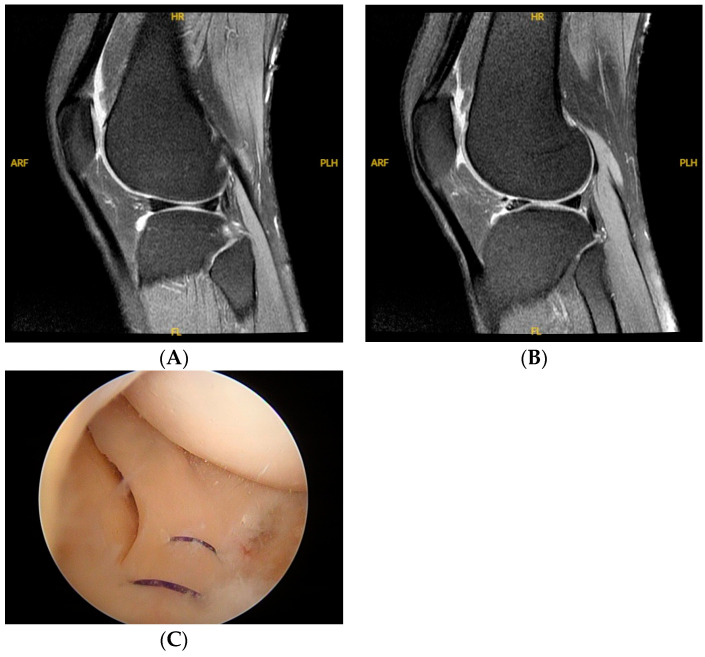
Preoperative knee magnetic resonance images of a 59-year-old female patient, and the arthroscopic image following meniscal stabilization. (A). The image shows the central region of the knee, which appears normal. The meniscus structure is intact, with uniform signal intensity and no abnormal high-signal areas. (B). The lateral side of the knee, where a hyperintense lesion is observed anterior to the meniscus. Additionally, there is fluid accumulation at the anterior horn, which may indicate synovitis or another inflammatory reaction. (C). Following meniscal stabilization.
